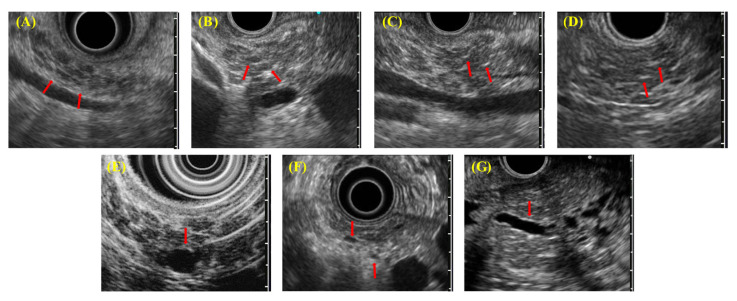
Each endoscopic ultrasound (EUS) finding in diagnostic criteria for early chronic pancreatitis 2009 (DCECP2009). (A) Lobularity with honeycombing; (B) lobularity without honeycombing; (C) hyperechoic foci without shadowing; (D) stranding; (E) cysts; (F) dilated side branches; (G) hyperechoic main pancreatic duct (MPD) margins.
